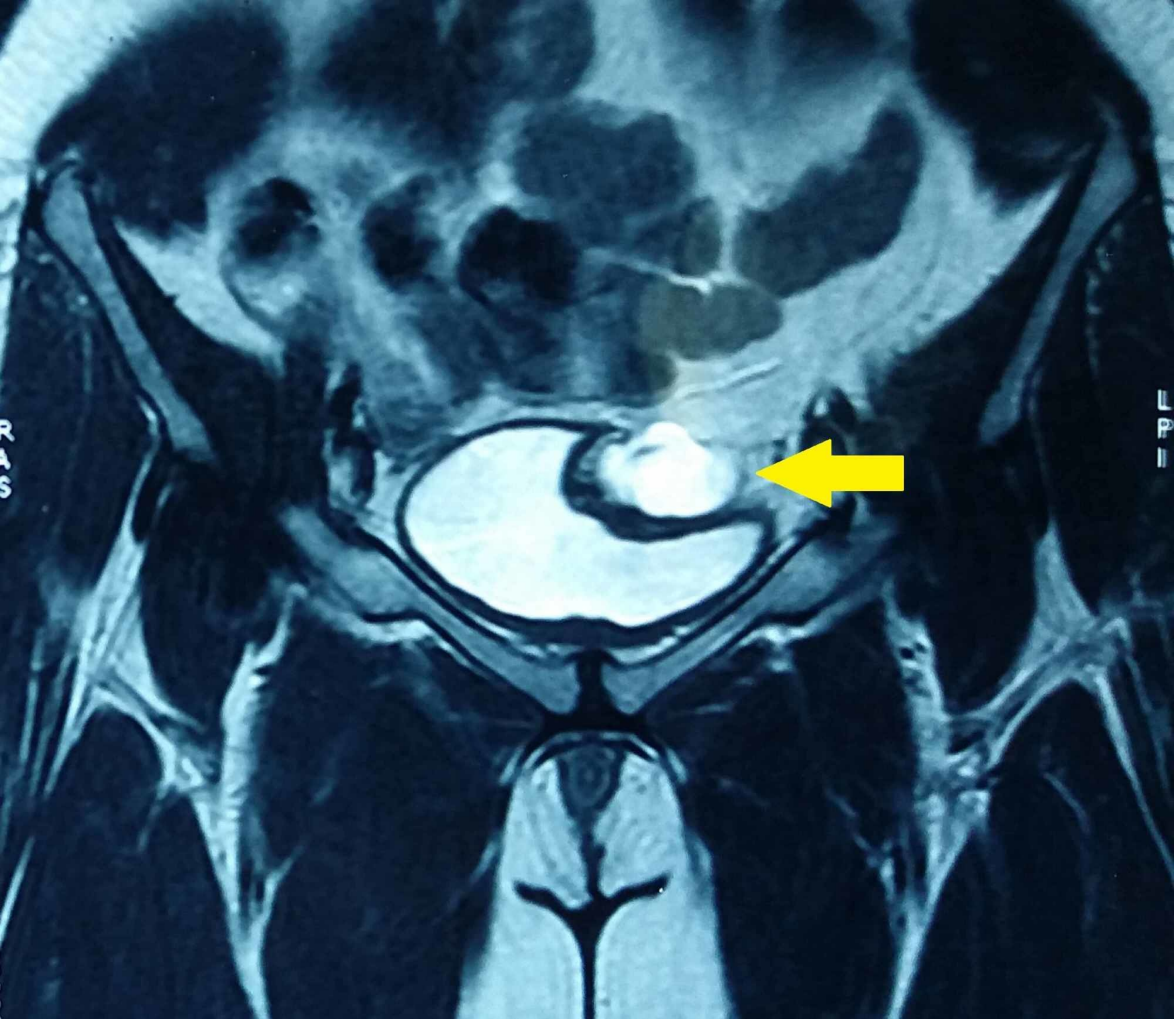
Coronal section of MRI depicting the hyperintense urachal cyst (arrow) in T2 sequence MRI: magnetic resonance imaging
